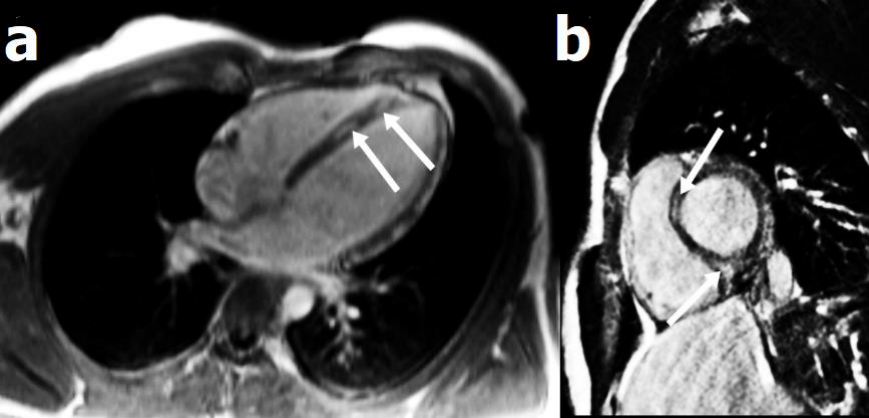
four-cavity (a) and short-axis (b) cardiac MRI showing late gadolinium enhancement in septal wall, suggestive of myocarditis
